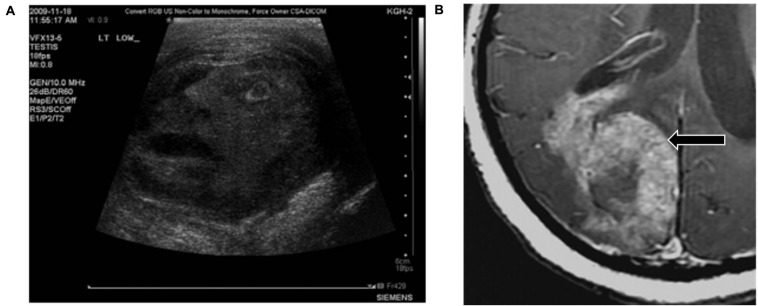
Examples of pareidolia that neither assist with nor hinder diagnosis. (A) The scrotal ultrasound from a patient with a testicular condition appears to resemble the face of a man in pain. (B) This lethal glioblastoma resembles a rabbit. Reproduced from Roberts and Touma (2011) and Massoud and Kalnins (2016), respectively.
